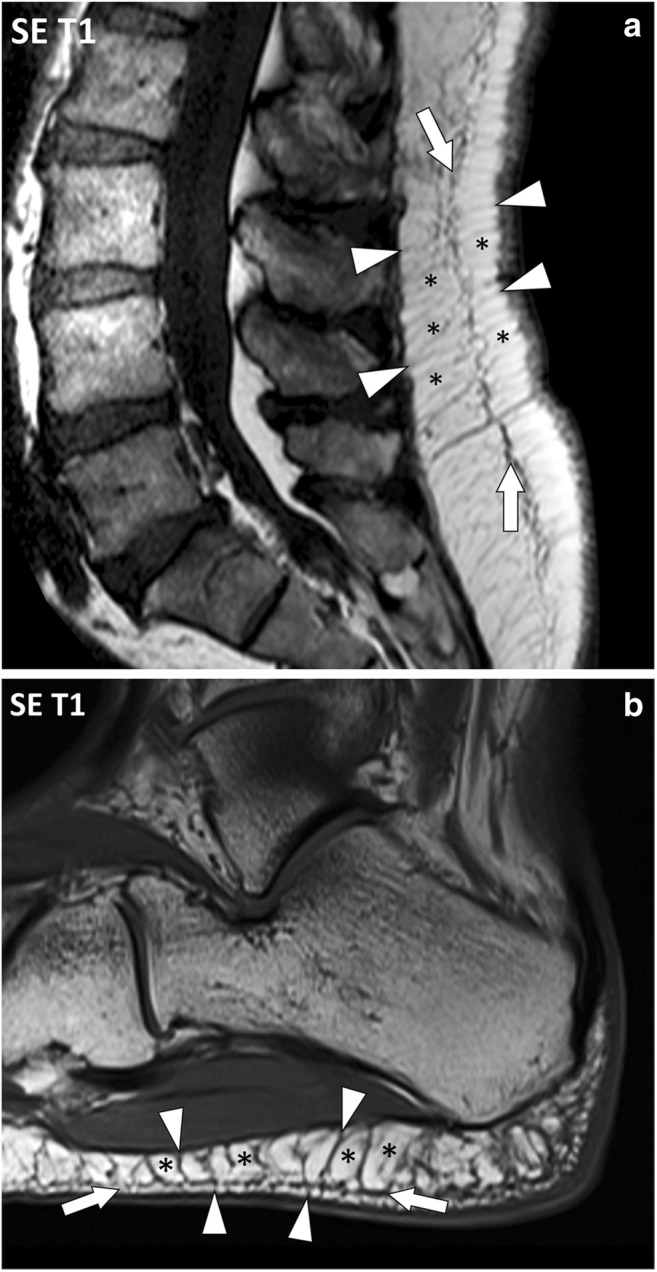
Sagittal SE T1-weighted images of (a) the lumbar spine and (b) the heel of two different patients. The fascia superficialis (arrows) is well depicted with fibrous tracts (arrowheads) perpendicular to the dermis and to the deep peripheral fascia, delineating fat lobules (asterisks). These attachments vary according to the considered body segment
